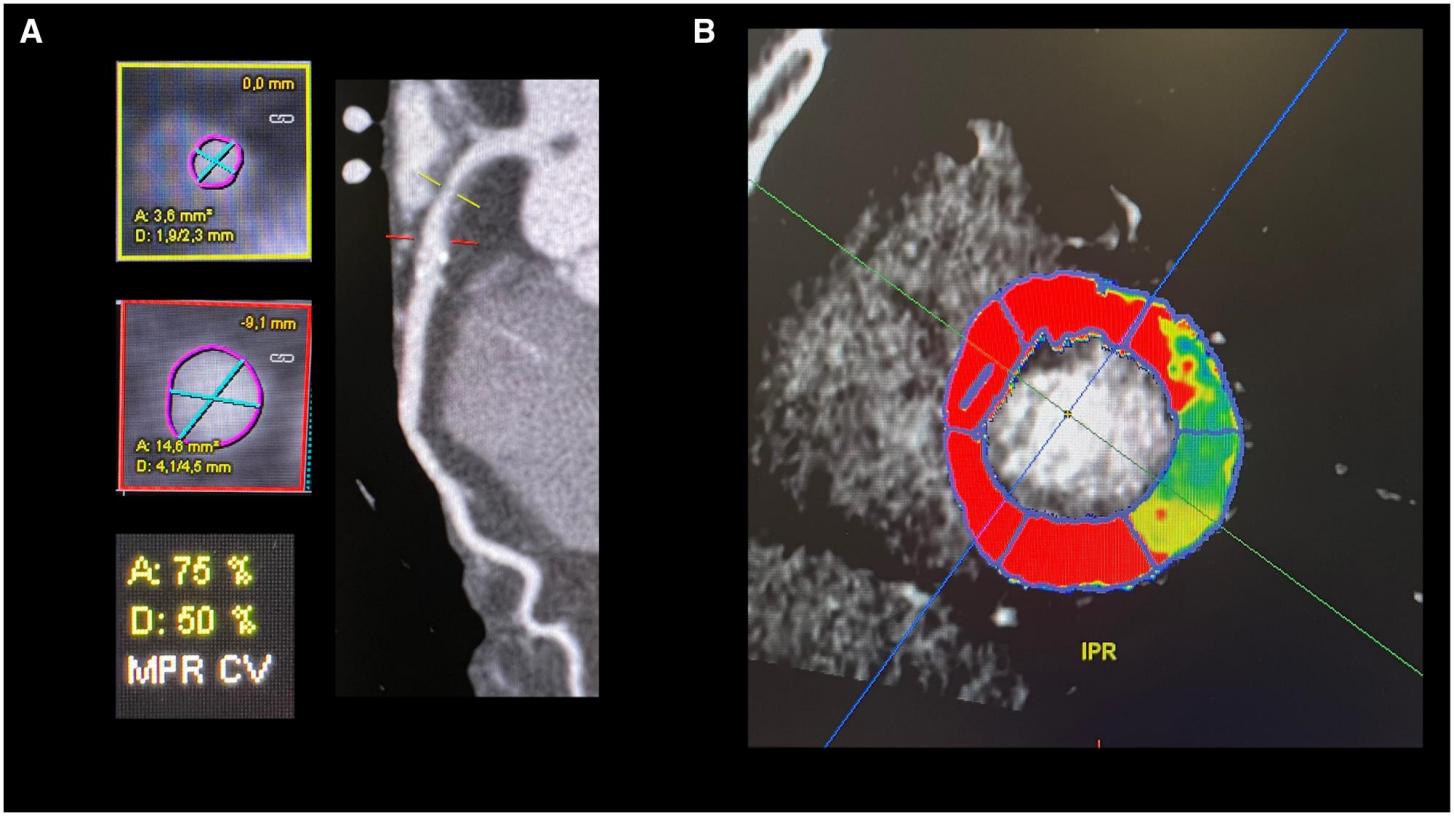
A man in his 70s undergoing investigation for suspected coronary artery disease. (A) Coronary computed tomography angiogram (CCTA) reveals a partially calcified plaque in the proximal segment of the left anterior descending artery (LAD), resulting in approximately 50% diameter stenosis, and 75% area stenosis of the lumen. Subsequently, the patient underwent dynamic computed tomography perfusion imaging, which, as shown in the short-axis plane (B), exhibited impaired perfusion in the corresponding myocardial segments. Abbreviations: MPR CV = multiplanar reformat of coronary vessel.
